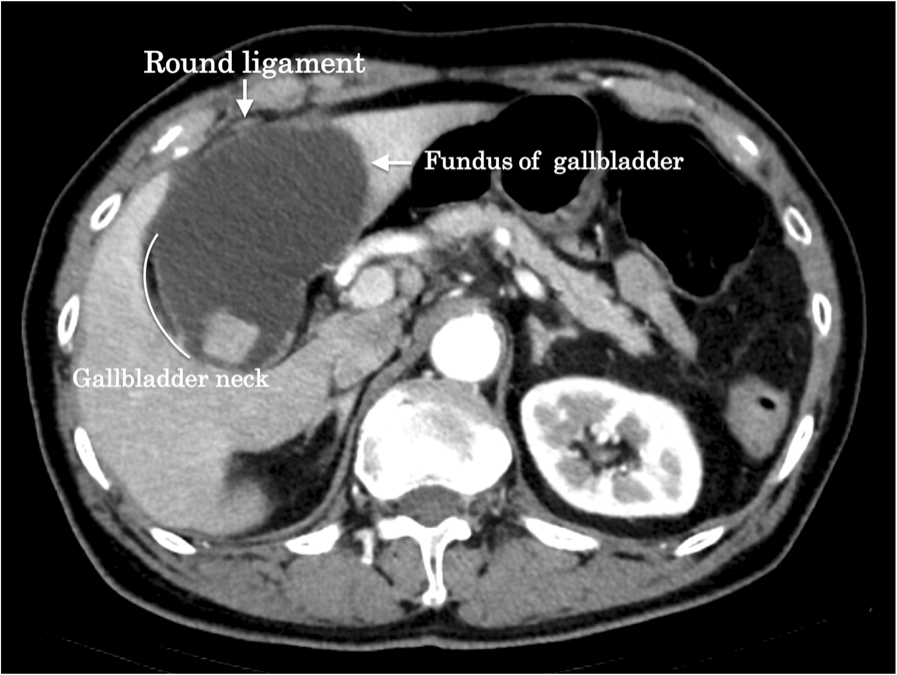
Abdominal contrast-enhanced computed tomography findings. The fundus of the gallbladder was located centrally beyond the round ligament (white arrow)
